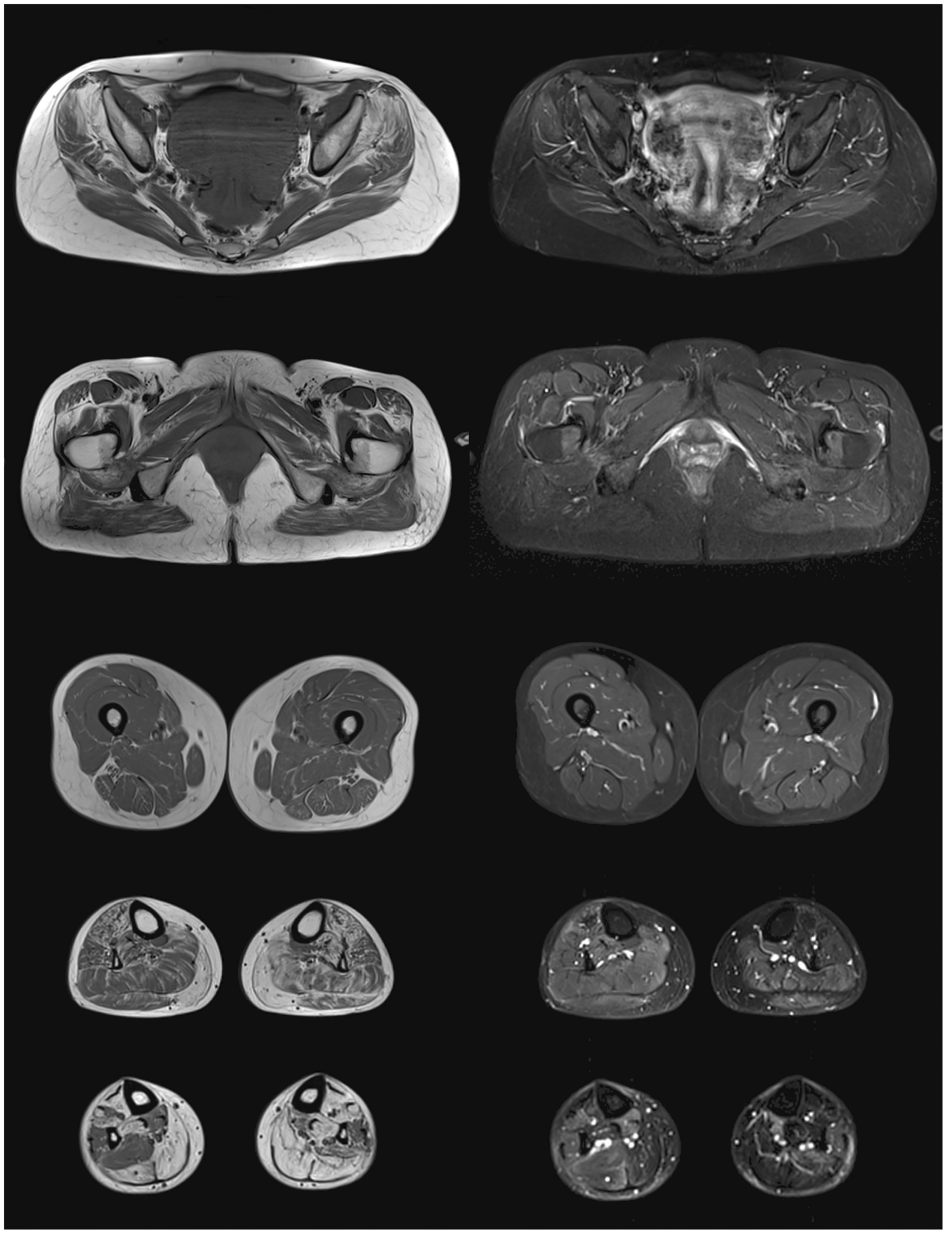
Lower body skeletal muscle MRI from the propositus. On the left panel, T1 images showing fatty degeneration as hyperintense signal in *gluteus minimus* and *medius, adductor maximus, quadratus femoris, tibialis anterior, gastrocnemius*, and *soleus*. On the right panel, STIR images showing interstitial edema as hyperintense signal in partially infiltrated muscles of the legs.
